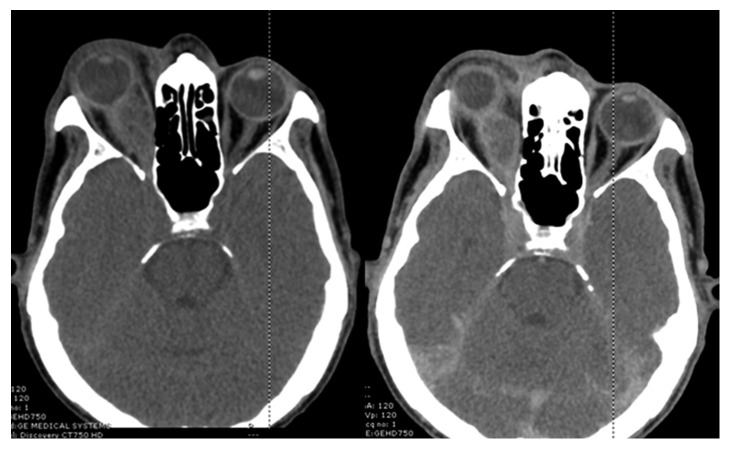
Computed tomography showing rim-enhancing hypodense lesion at the right orbital apex abutting to the optic nerve sheath complex and right medial rectus muscle.
